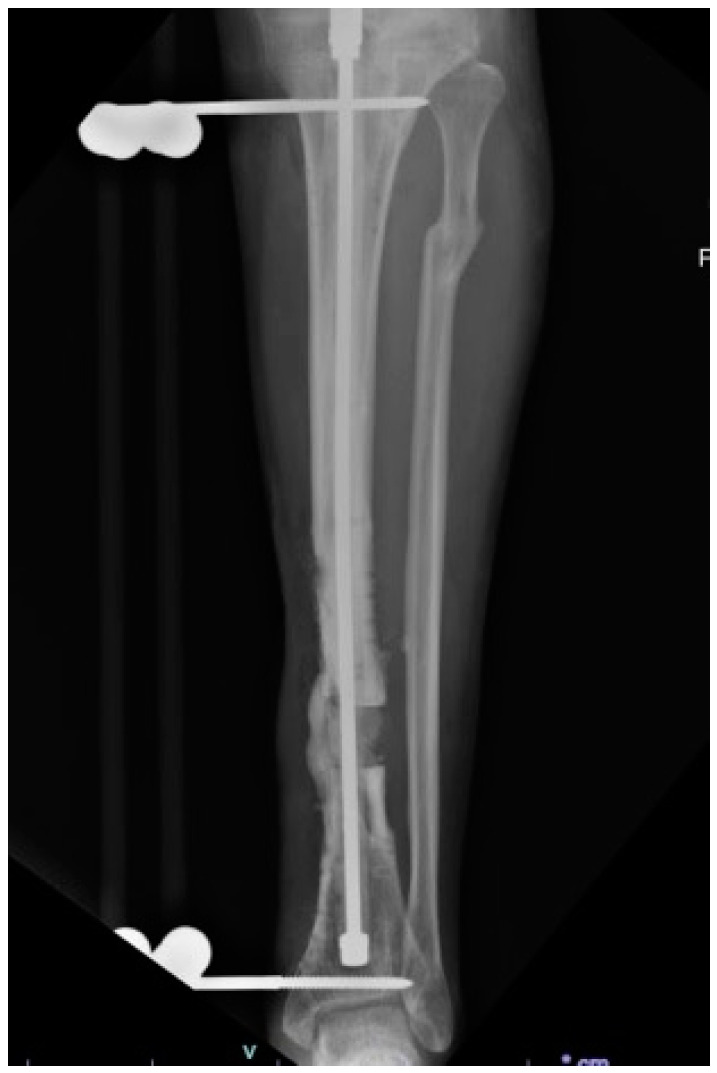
Radiograph of a tibial critical-sized bone defect associated with a fracture nonunion complicated by infection. The patient status is post-placement of an external fixator with an intramedullary antibiotic device pending distraction osteogenesis (Radiographs courtesy of Dr Joseph Patterson).
